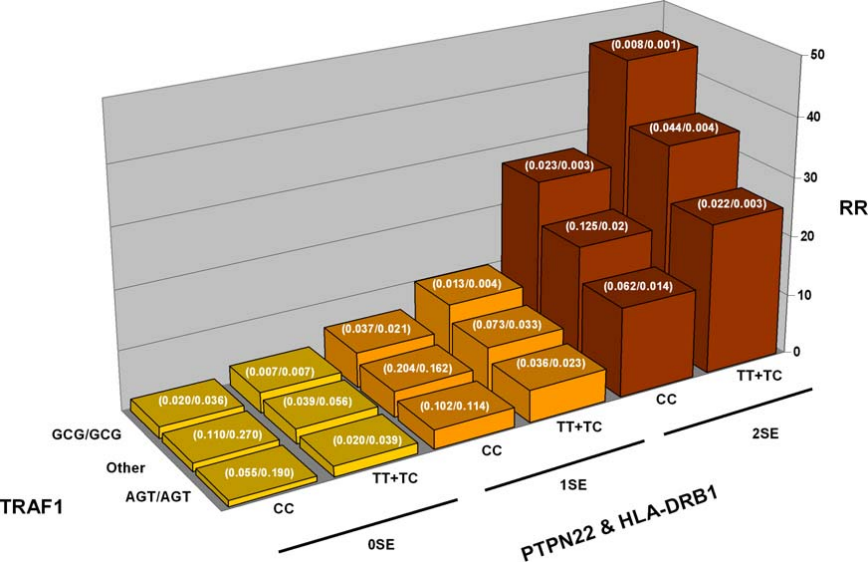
Relative risk plotted as a function of the genetic load of three validated RA risk variants in *HLA-DRB1*, *PTPN22* and *TRAF1*. Individuals are classified according to the number of copies of the *HLA-DRB1* shared epitope (0, 1 and 2) (SE-positive *HLA-DRB1* alleles found in this sample set were: 0101, 0102, 0401, 0404, 0405, 0408 and 1001), carriage of the W620 *PTPN22* missense SNP (TT + CT vs CC) and diplotypes at the TRAF1 SNPs, rs2239657, rs7021880 and rs7021049. The frequency of each combination of markers in cases and controls is highlighted in white (case/control).
